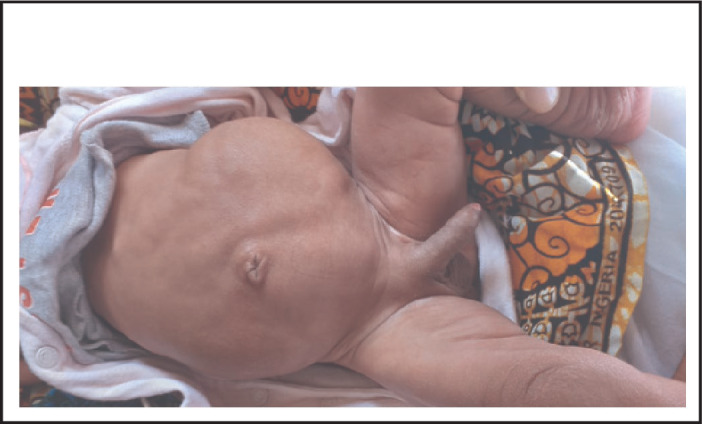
Laboratory investigations (Table 1) revealed increased creatinine level. Urinalysis showed pale yellowish urine, with pus cell 0-2/Hpf, RBCs >100Hpf. His complete blood count was normal. Abdominal ultrasound showed bilateral hydronephrosis (Figure 9).
